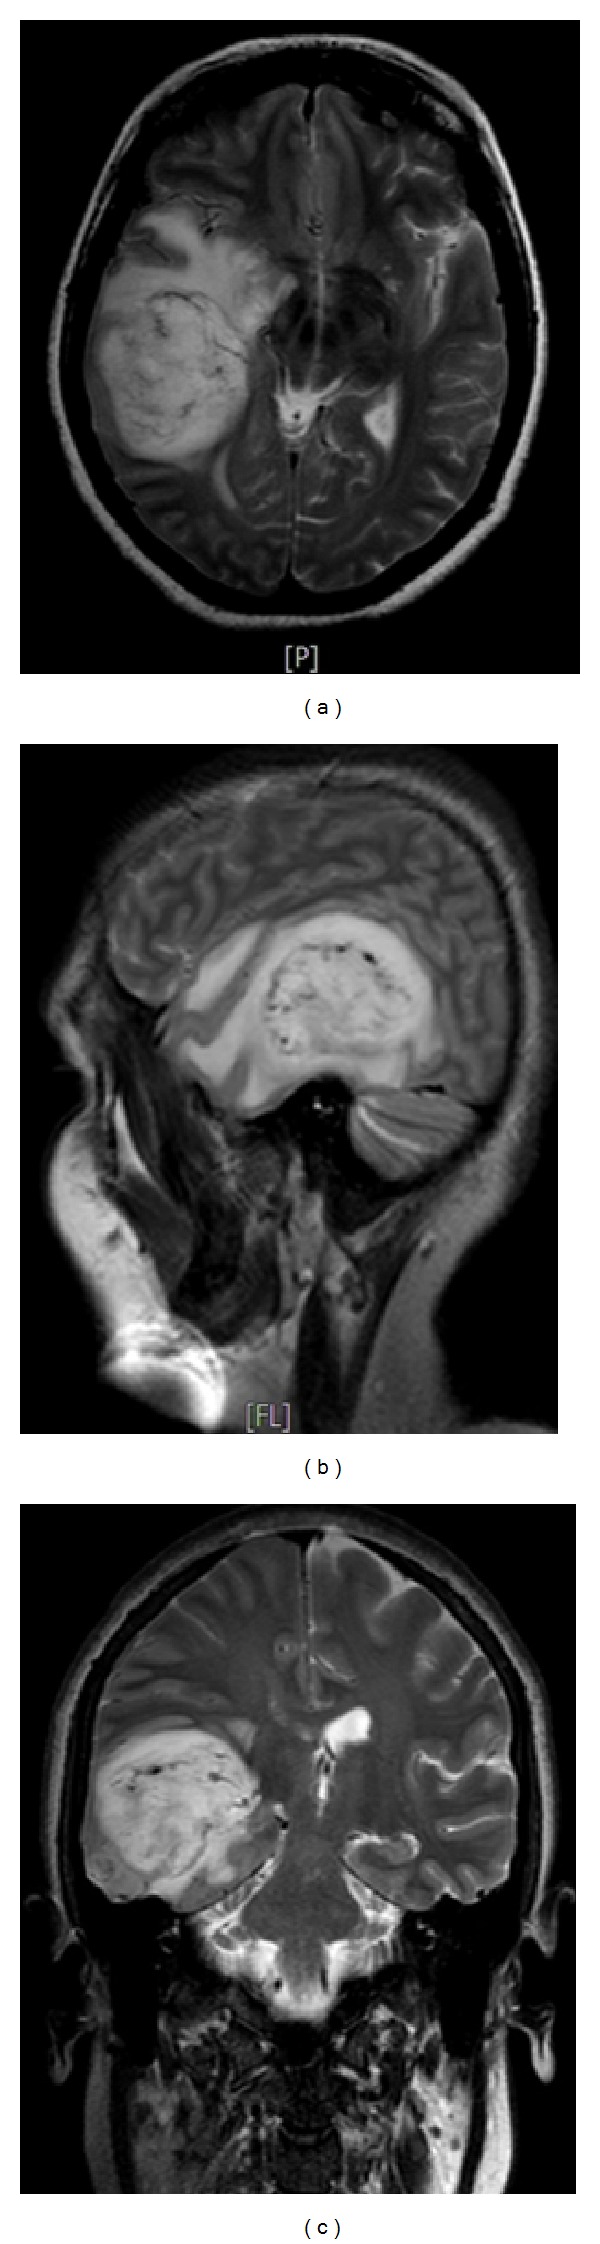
T2 weighted MR images on axial (a), sagittal (b), and coronal planes (c) show heterogenous hyperintense mass lesion on right temporooccipital lobe with prominent peripheral vasogenic edema.
